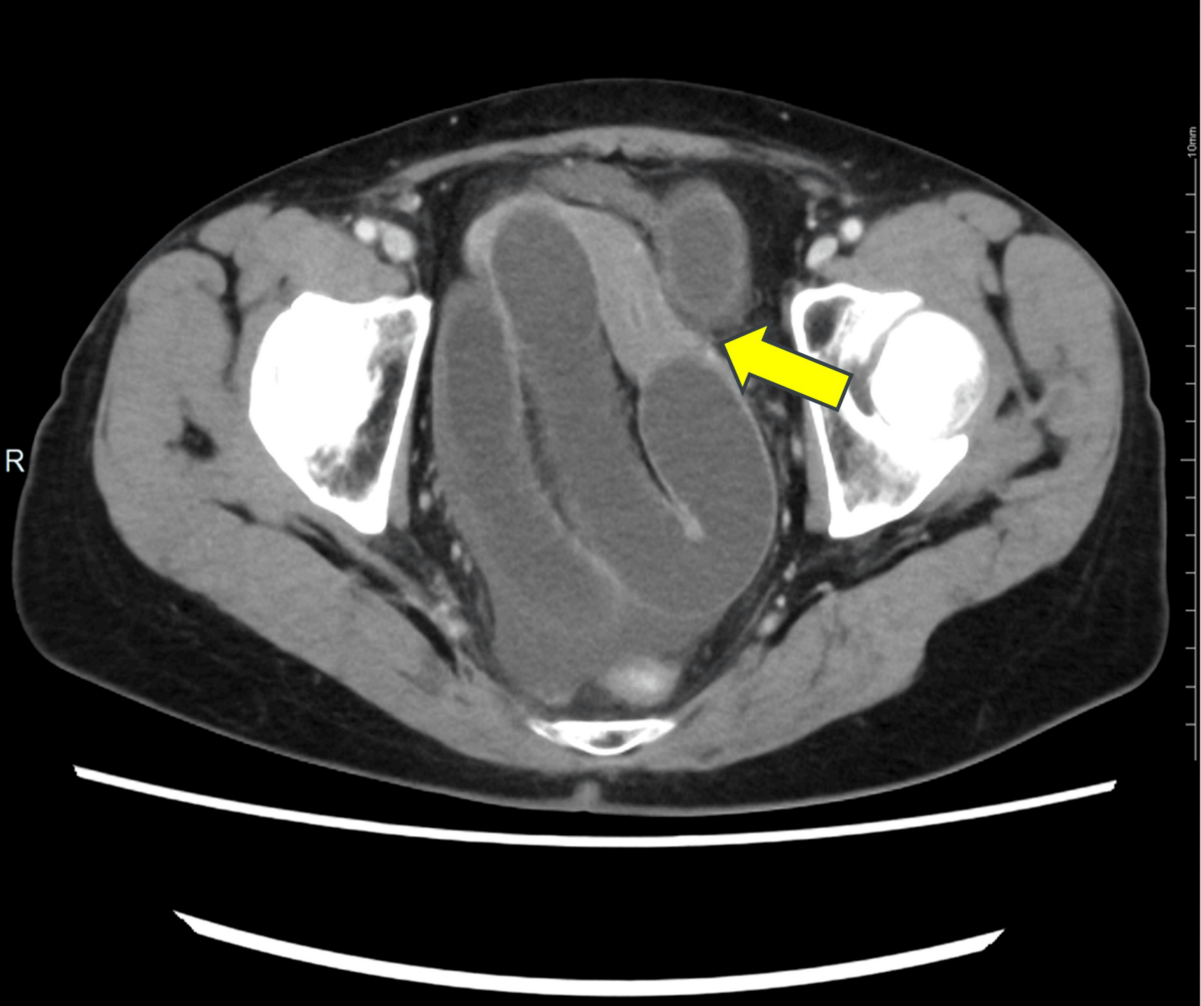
Contrast-enhanced abdominal CT revealed an ileal loop within the Douglas pouch, leading to anterior and rightward displacement of the uterus.
